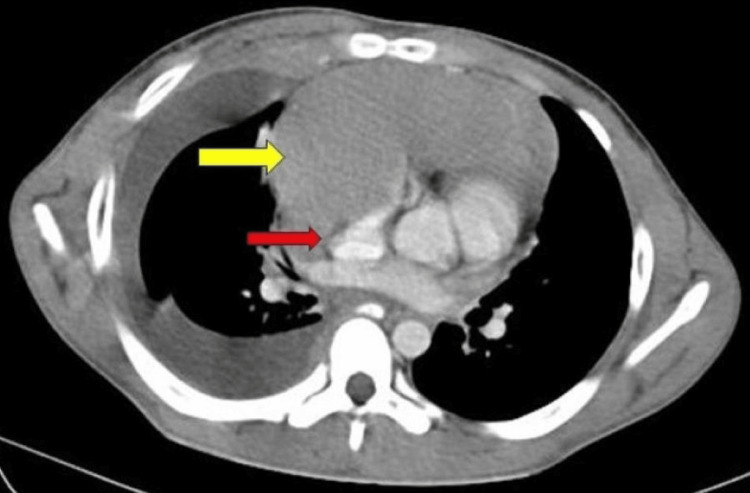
Contrast-enhanced computed tomography of the thorax showing mediastinal mass (yellow arrow) compressing the superior vena cava (red arrow).
